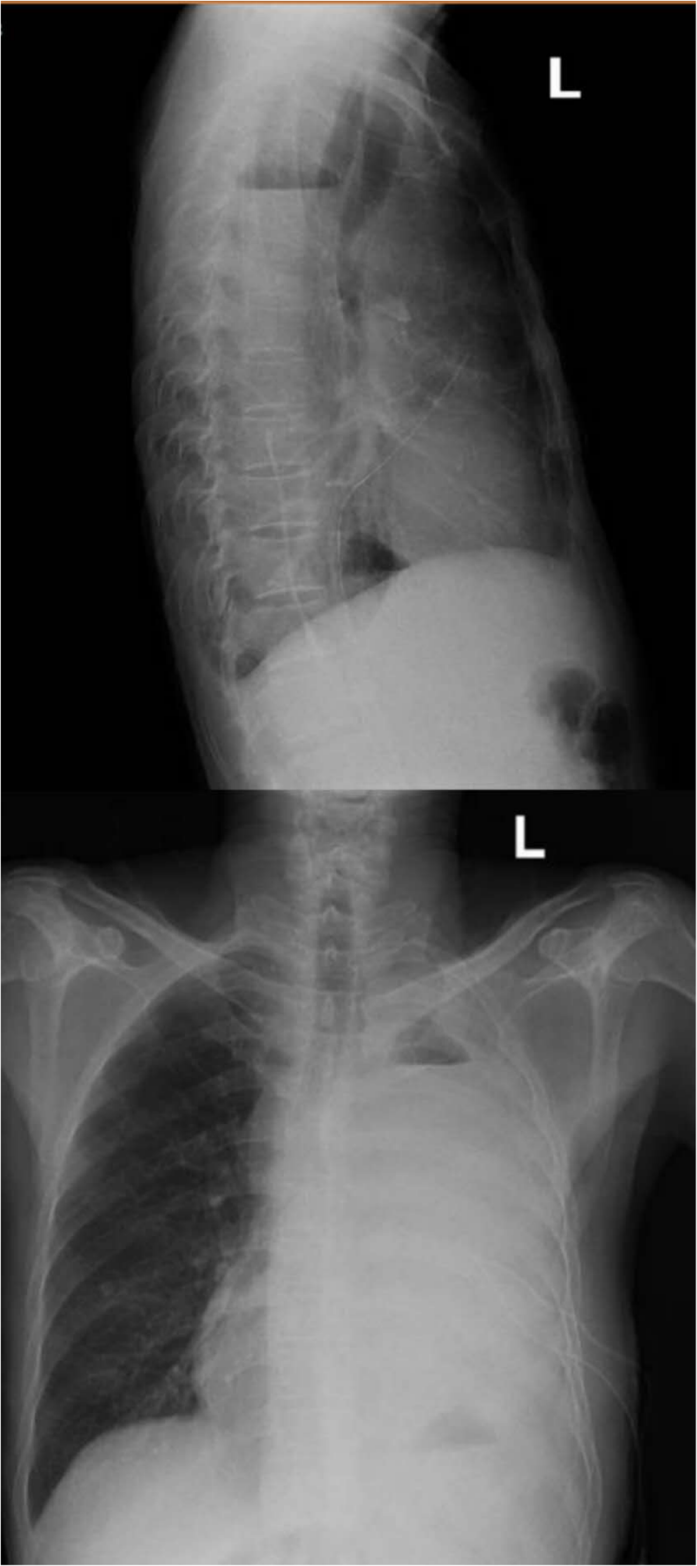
Chest X-ray image after the patient underwent closed thoracic drainage. It showed extensive hydropneumothorax (massive pleural effusion) with atelectasis
